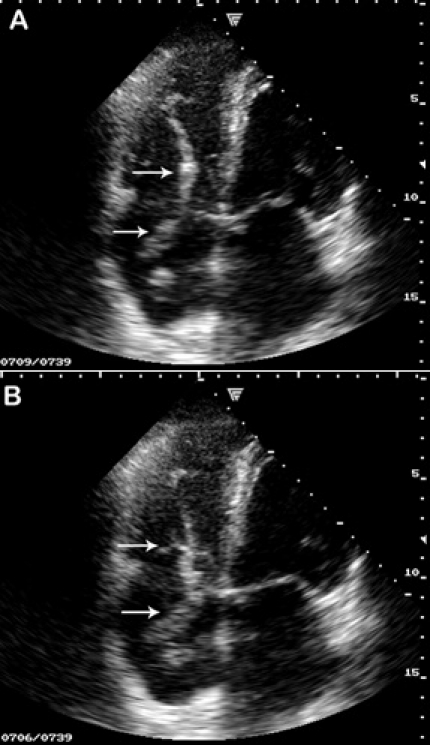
Two-dimensional transthoracic echocardiography
